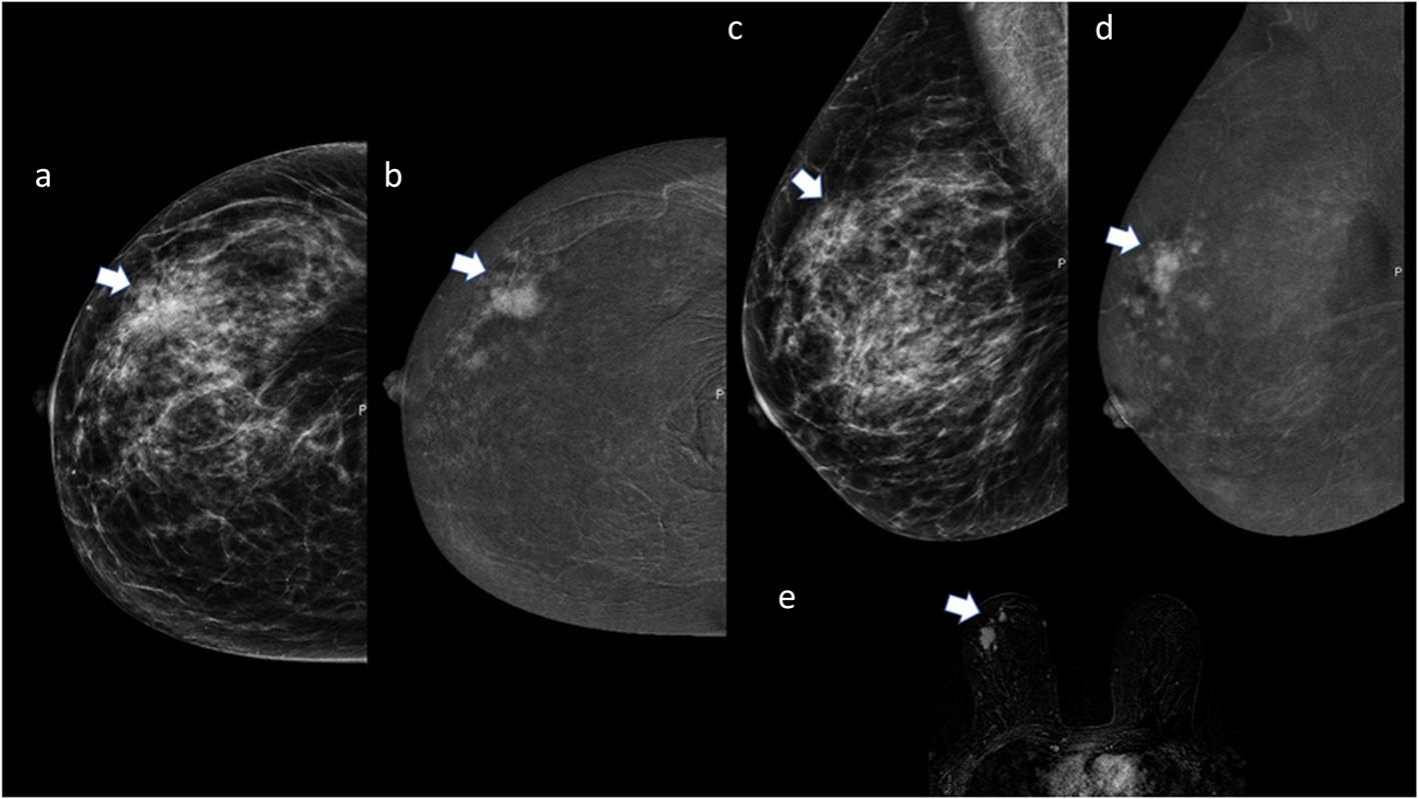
Typical presentation of invasive lobular carcinoma on CEM and comparison with breast MRI
